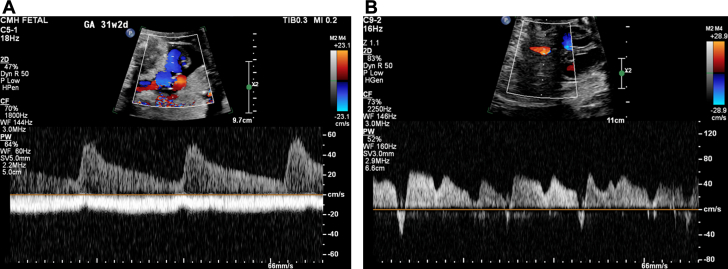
Abnormal Fetal Umbilical and Ductus Venous Doppler Waveforms (A) Umbilical venous (UV) Doppler below the baseline demonstrating UV pulsations. Normal umbilical arterial Doppler above the baseline aside from fetal bradycardia. (B) Abnormal ductus venosus Doppler pattern with a-wave reversal.
